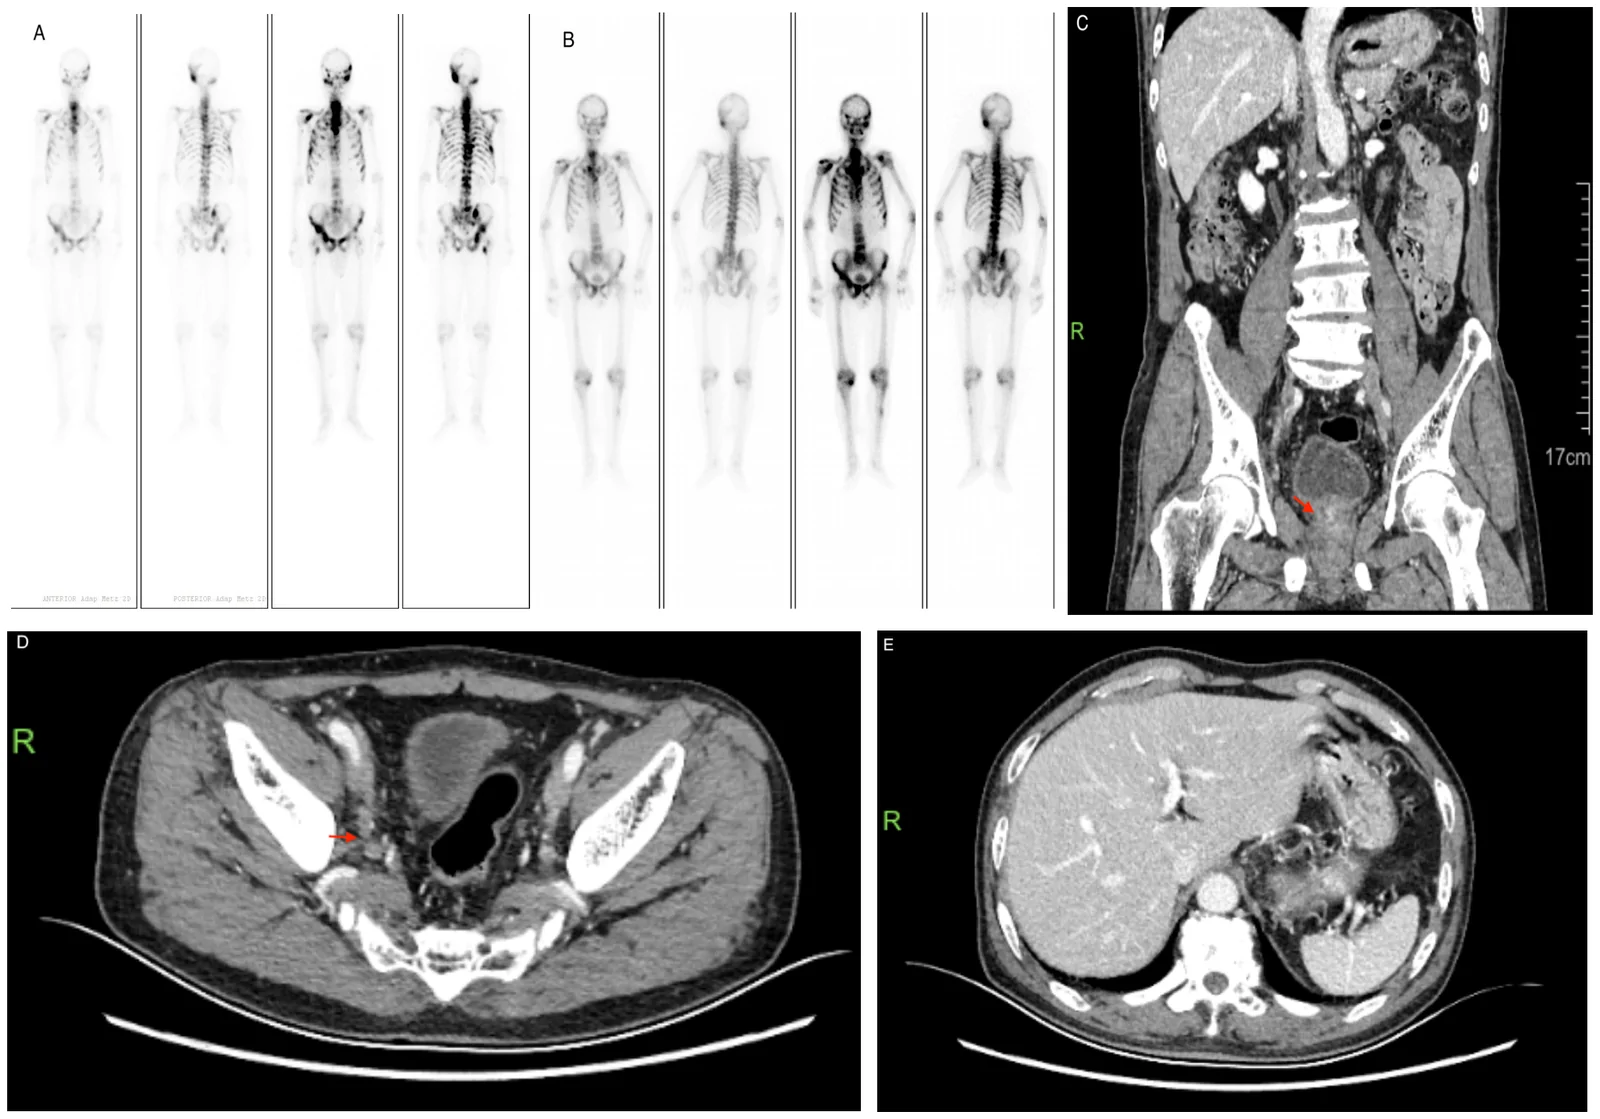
(A) Pre-treatment ECT (2025.9.23): Extensive areas of increased radiotracer uptake throughout the axial and appendicular skeleton, consistent with multifocal osteoblastic bone metastases. (B) Post-treatment ECT (2026.4.21): Decreased intensity and slight reduction in the extent of bone metastatic lesions compared with prior imaging. (C–E) Contrast-enhanced abdominal CT (2026.4.23): (C) Irregular prostatic morphology with indistinct boundaries relative to the rectum. (D) Pelvic lymph nodes with indistinct margins. (E) Liver with no evidence of metastatic lesions.
